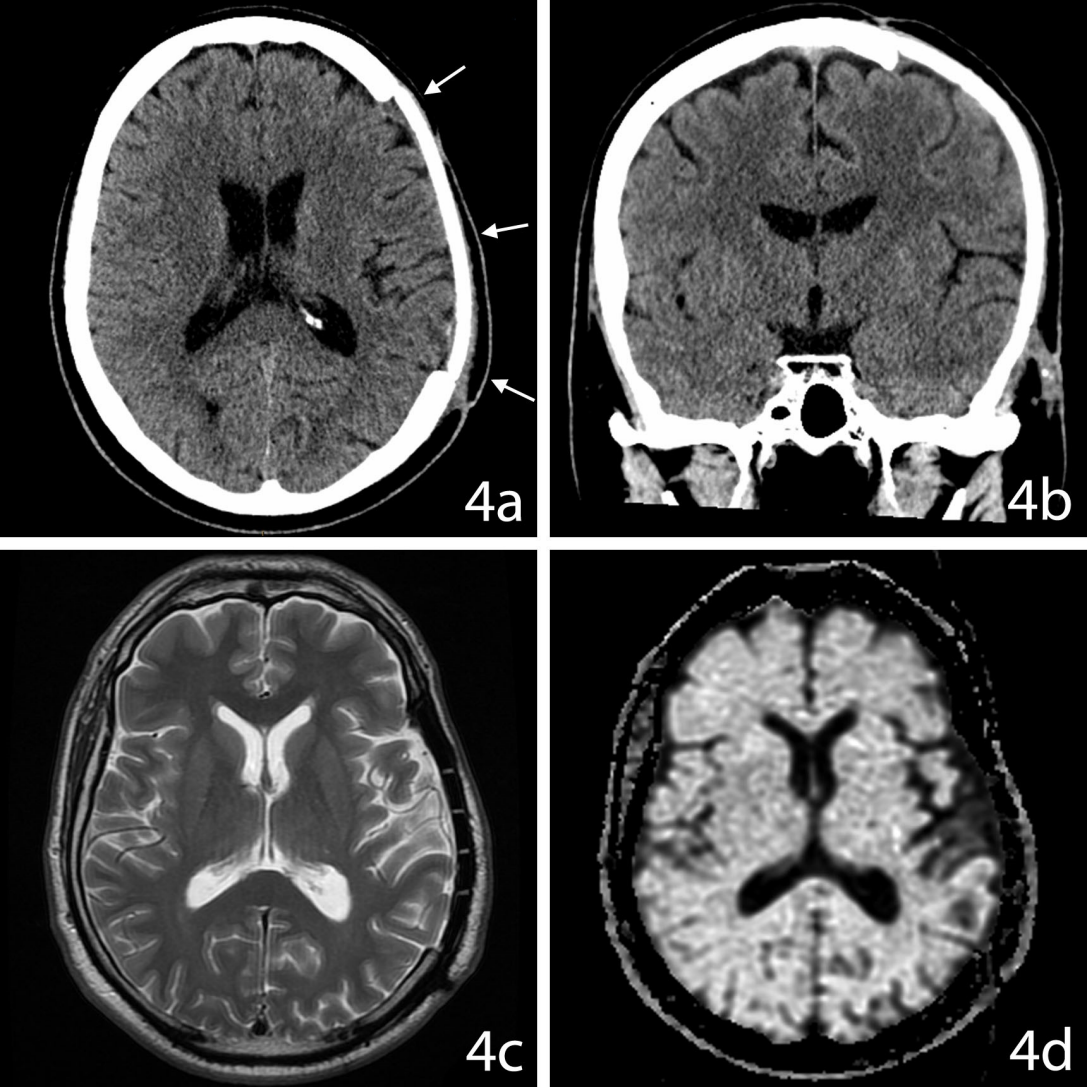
15-Month Follow-Up Imaging Aggressive osteoblastoma imaging after surgical resection and at 15 months follow-up. 4a: Axial CT. 4b: Coronal CT. 4c: Axial T2-weighted MR. 4d: Axial T1-weighted MR. The synthetic bone flap is evidenced by the white arrows (4a) on CT, which didn’t show signs of recurrent lesion (4b). A diffuse hyperintense signal is observed within the periventricular white matter on T2-weighted MR (4c). The signal changes are not from a discrete lesion, but rather vague areas of increased signal. T1-weighted MR (4d) demonstrates low intensity in the left parietal region when compared to adjacent normal cortex, consistent with encephalomalacia. There is no change in ventricle size to suggest that the signal change is related to either gliosis or mass lesion.
